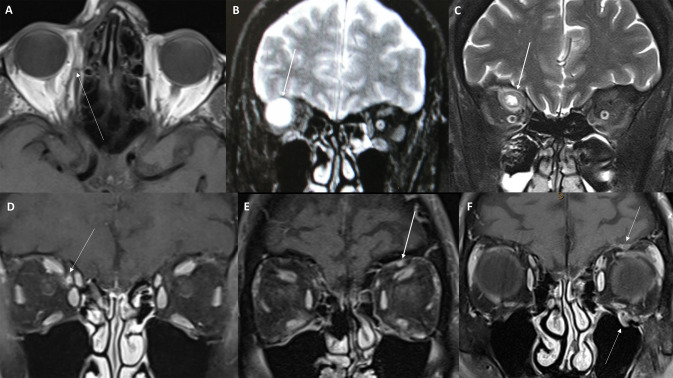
MRI findings in periocular squamous cell carcinoma with perineural spread. A Axial T1-weighted sequence demonstrates thickening of the right nasociliary nerve and anterior ethmoidal nerve (arrow). B Perineural spread in the orbit can manifest as a cystic mass with a hyperintense T2 signal (arrow), which may be representative of hypercellularity secondary to neoplastic infiltration. C T2 coronal sequence demonstrating right frontal nerve enhancement and enlargement. D–F Coronal T1 fat-suppressed contrast-enhanced sequences demonstrating different examples of V1 and V2 perineural spread with enlargement and enhancement of the nasociliary nerve (D), frontal nerve (E, F) and infraorbital nerve (F).
